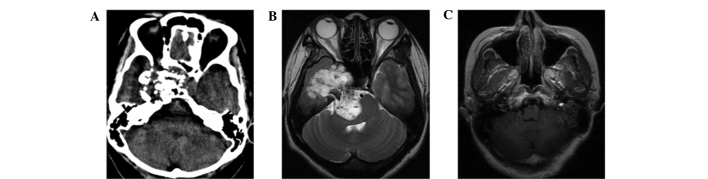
Pre-operative images showing lesions located in the parasellar region, the middle and posterior cranial fossa and the jugular foramen region, respectively. (A) Contrast-enhanced CT scan showing a mass lesion located in the right parasellar region. (B) T2-weighted MRI scan showing a mass lesion extending from the middle fossa to the posterior cranial fossa. (C) T1-weighted MRI scan with contrast showing an enhancing mass in the left jugular foramen region. CT, computed tomography; MRI, magnetic resonance imaging.
